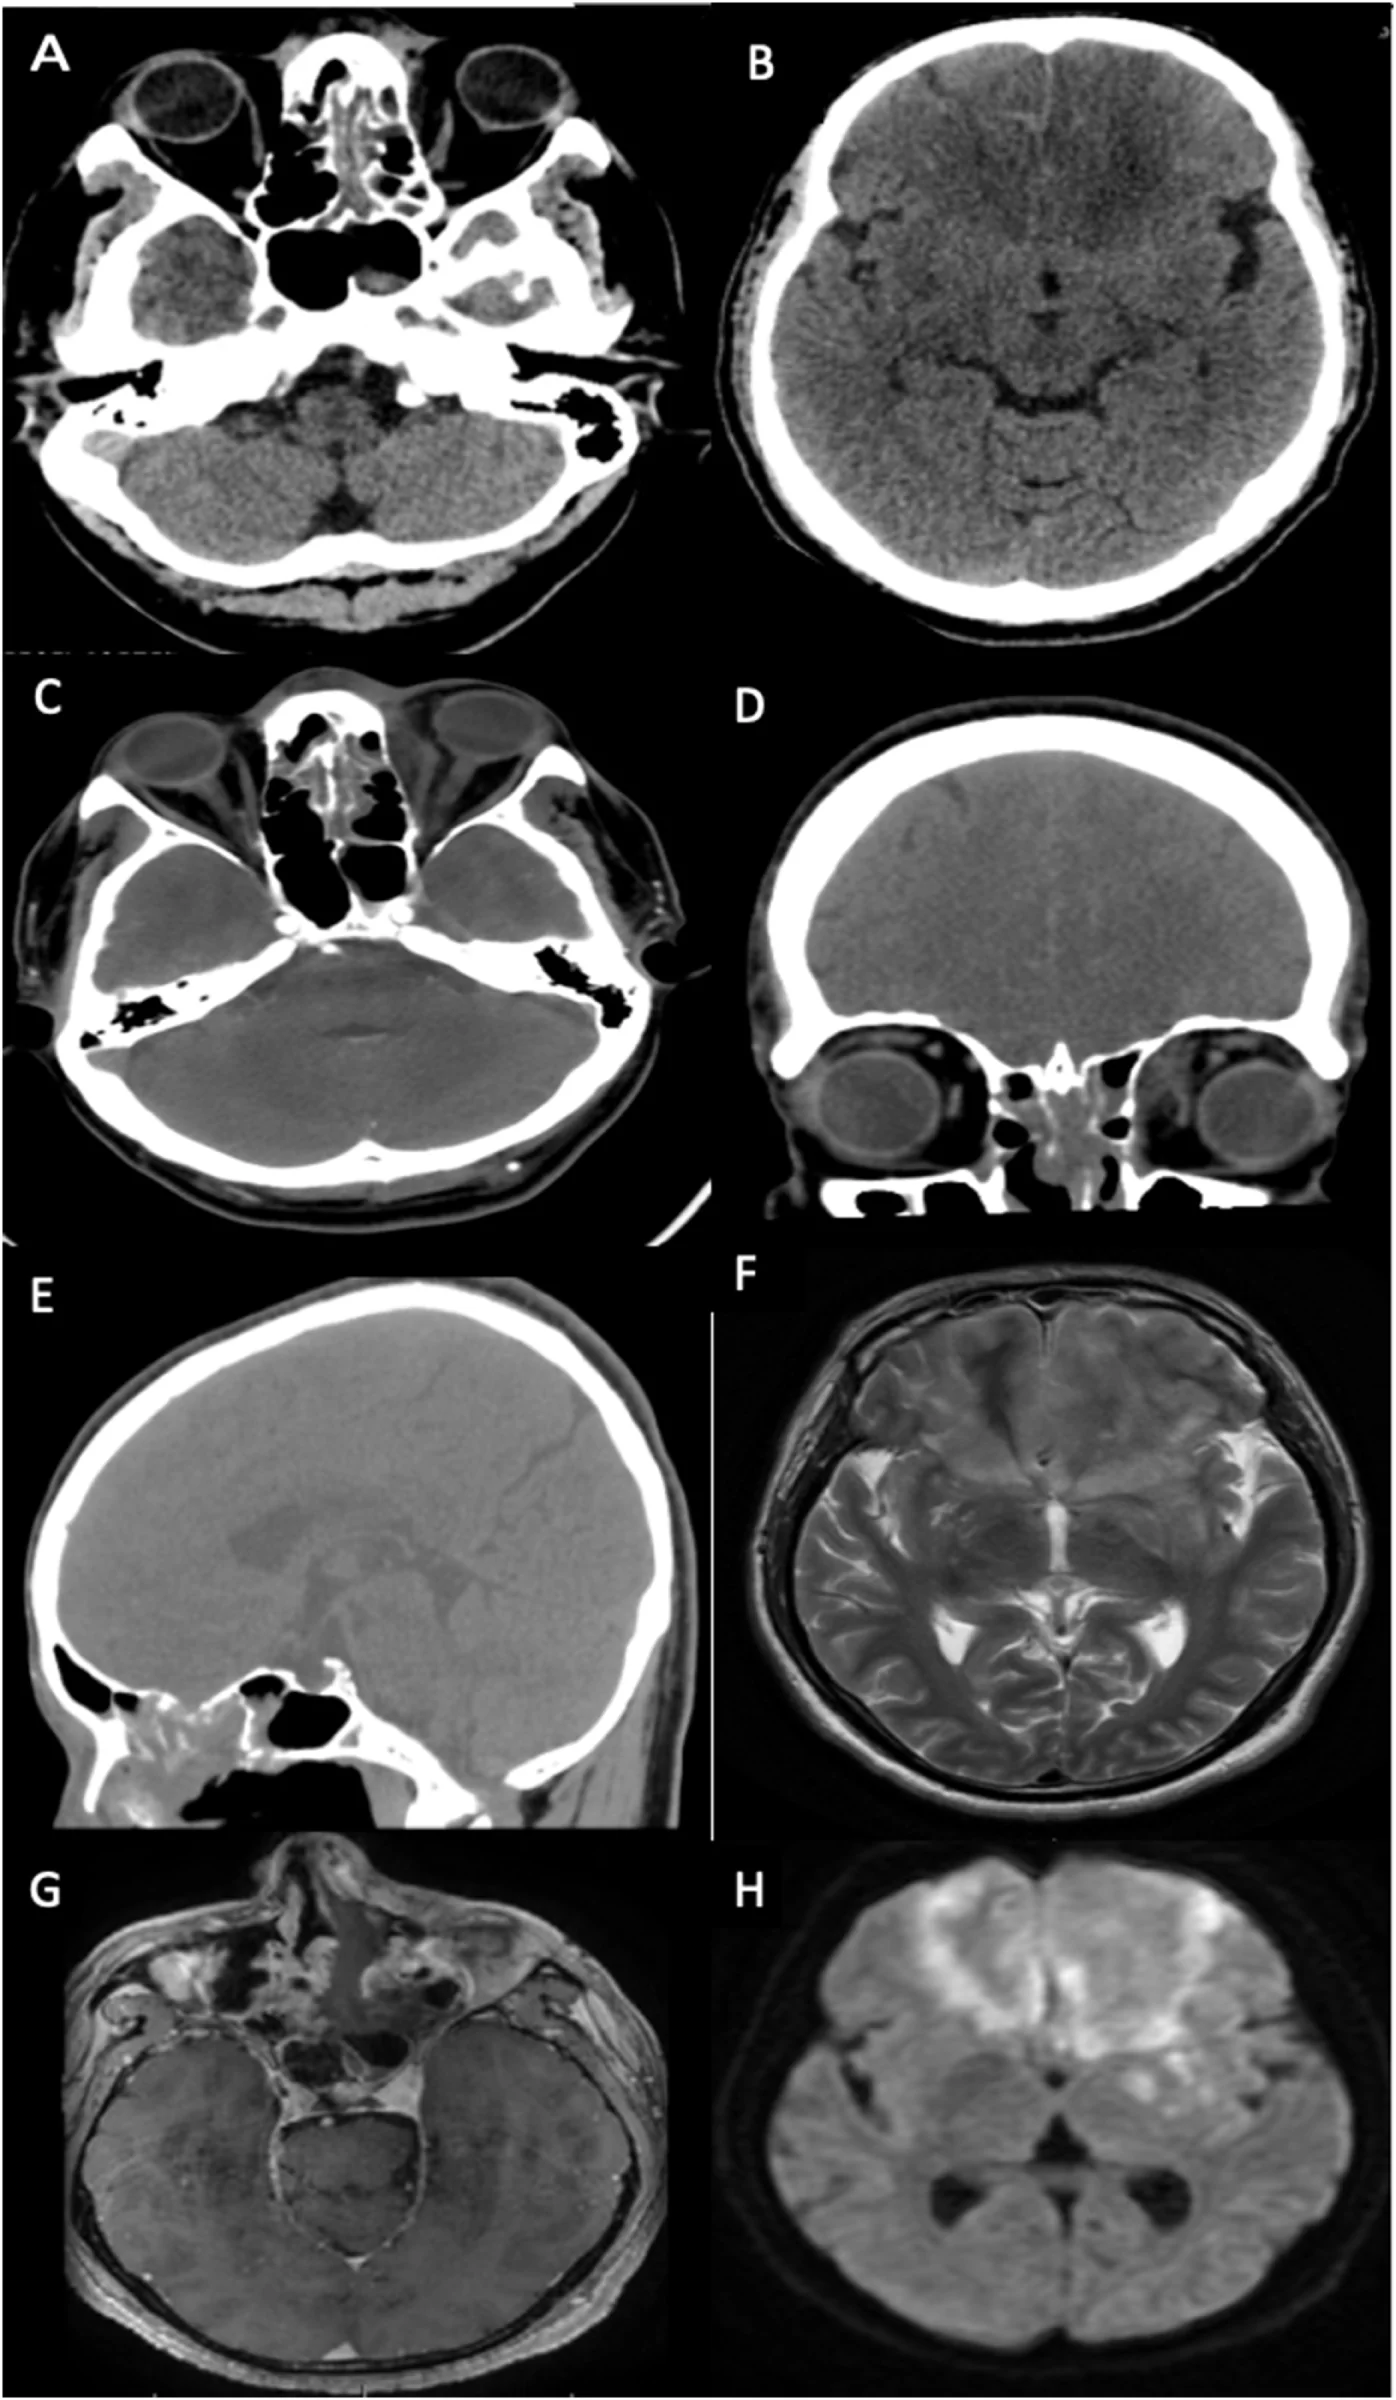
Non-enhanced brain CT scans, soft-tissue axial views. (A) Opacification of the left frontal sinus, sphenoid sinus, and ethmoid air cells. (B) Bifrontal ill-defined hypodensities extending anteriorly and laterally, producing a mild mass effect with an early left-to-right midline shift. Bone window images: (C) axial view, (D) coronal view, and (E) sagittal view, showing homogeneous opacification of the left frontal sinus, sphenoid sinus, and ethmoid air cells with hyperdense calcifications. There is an erosion of the lamina papyracea (medial orbital wall) on the left side and an extension of fungal elements into the medial extraconal orbital space. The left optic canal exhibits signs of thinning and partial dehiscence, indicating involvement of the optic nerve. The left orbital fat appears less well-defined and strandy, indicating orbital cellulitis. Magnetic resonance imaging (MRI) of the brain with contrast, axial views. (F) T2W image showing hyperintense edematous changes in the bilateral frontal lobes and left basal ganglia with mass effect on the frontal horns of the lateral ventricles. (G) T1W post-contrast image showing left medial orbital intraconal intermediate signal intensity with no definite contrast enhancement. There is evidence of extension into the left cavernous sinus and adjacent brain parenchyma, involving the left medial temporal lobe and basal frontal lobe. Mucosal thickening of the left concha, left ethmoid, and left frontal sinuses is also noted. (H) DWI image showing diffusion restriction consistent with invasive fungal encephalitis with venous infarction due to cavernous sinus thrombosis.
